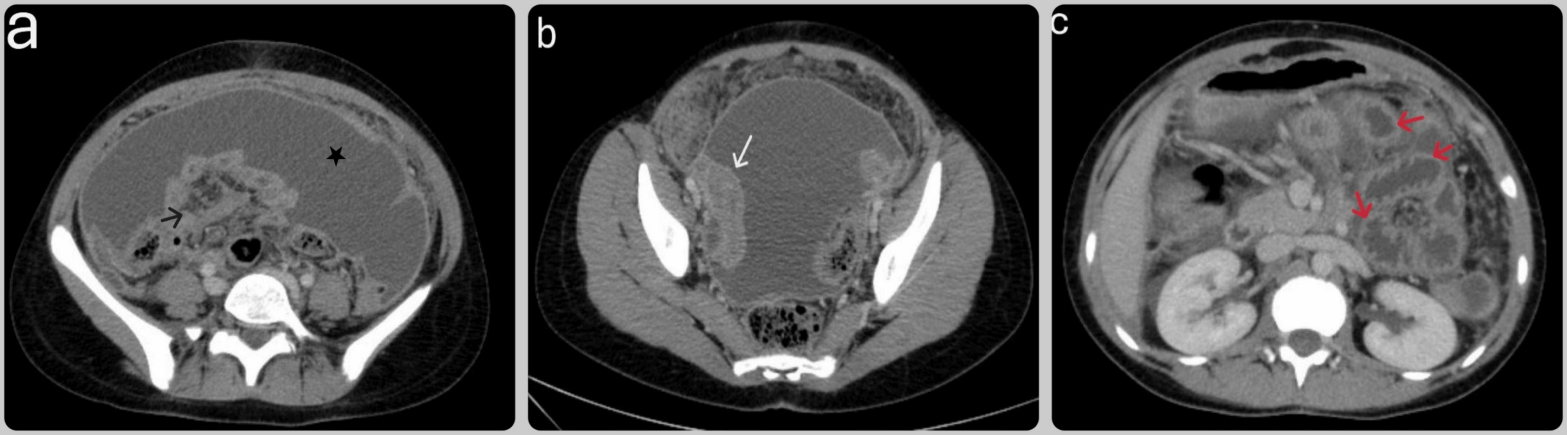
Abdomen CT scan (a) Axial CT scan of the abdomen showing diffuse nodular thickening of the mesentery (black arrow), with a thickened and enhanced peritoneum. Extensive ascites are visible as large fluid collections surrounding the abdominal organs (black star). (b) Axial CT scan of the pelvis demonstrating thickened and enhanced fallopian tubes (white arrow), raising the possibility of salpingitis. (c) CT scan image displaying fluid-filled, prominently congested jejunal loops (red arrows).
